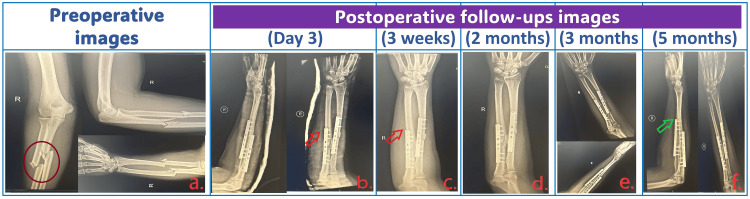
Preoperative and postoperative radiographic follow-up images. a. Preoperative radiographic images showing comminuted fractures of both right forearm bones. b. Postoperative radiographic images (on POD3) showing increased soft tissue swelling around the operated site. c. Postoperative follow-up radiographic images at three weeks. d. Postoperative follow-up radiographic image at two months. e. Postoperative follow-up radiographic image at three months. Images c-e show decreased soft tissue swelling around the operated site. f. Postoperative follow-up radiographic images (at five months) showing normal soft tissues without swelling around the operated site. POD: postoperative day; dark brown circle in (a): involved fractured site of the right forearm; red arrow: soft tissue swelling around the operated site; green arrow: showing normal soft tissues (without swelling) around the operated site
